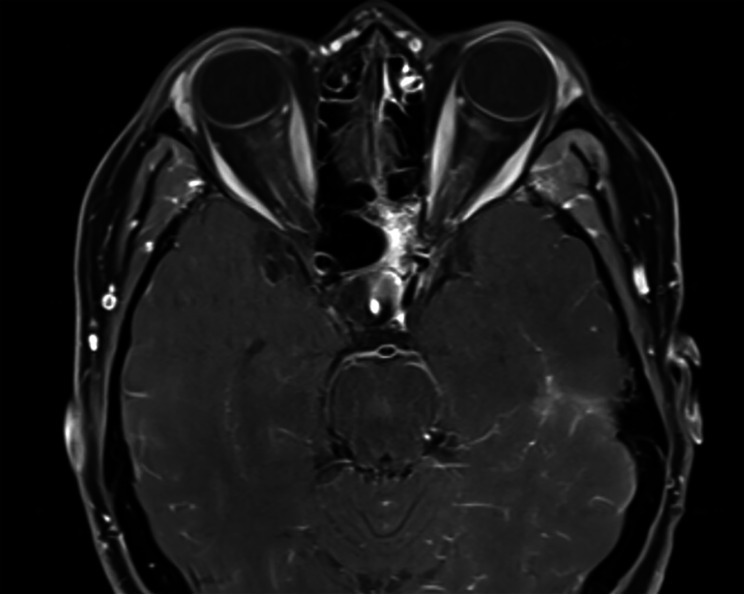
Axial T1 fat-suppressed magnetic resonance imaging shows optic nerve sheath enhancement bilaterally
